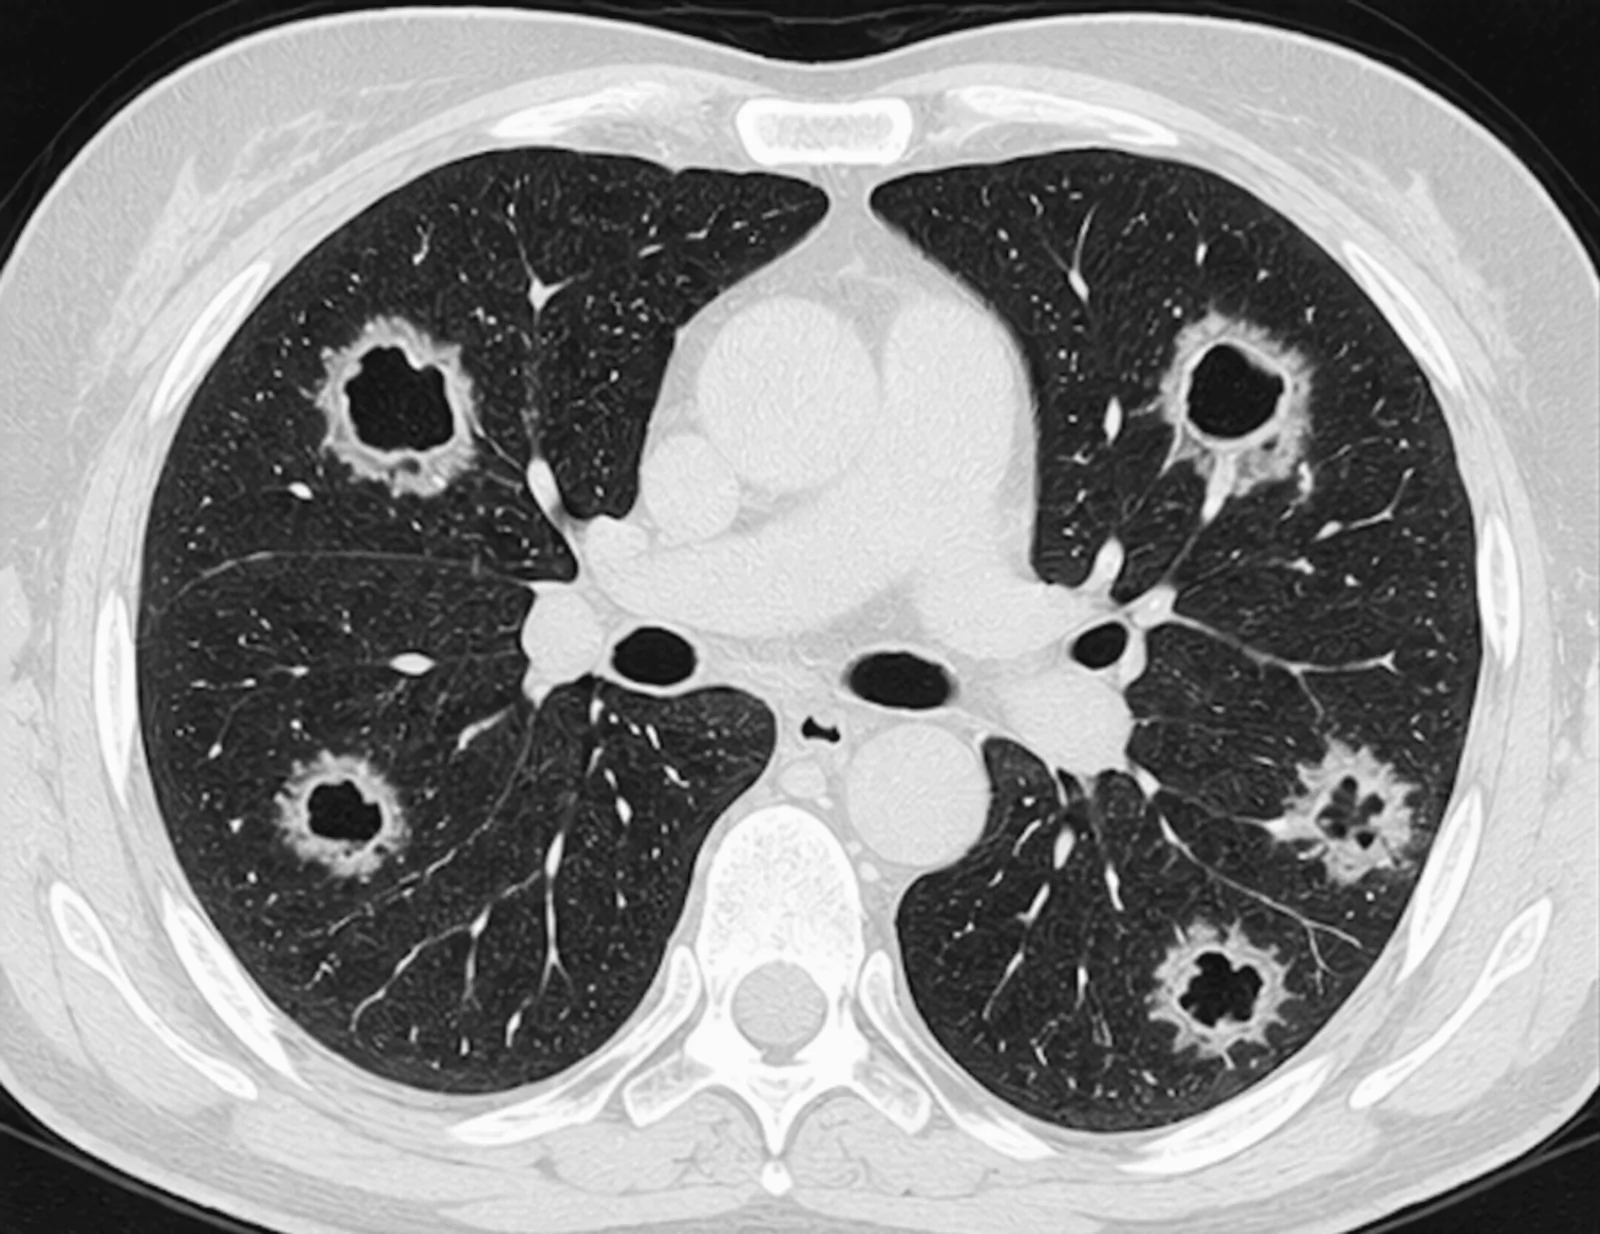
Computed tomography of the chest Computed tomography of the chest showed multiple bilateral cavitary pulmonary nodules of varying sizes with thick, irregular walls without evidence of mediastinal lymphadenopathy or pleural effusion.
